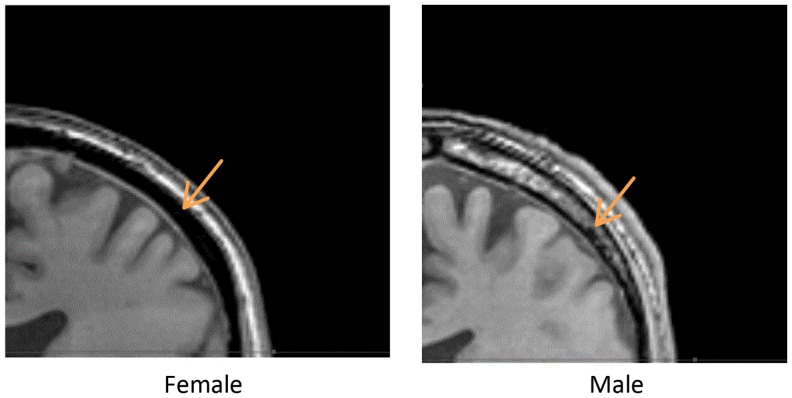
Above are two parietal coronal MRI sections through male (right) and female (left) heads. The arrows point to the cancelous bone region.
